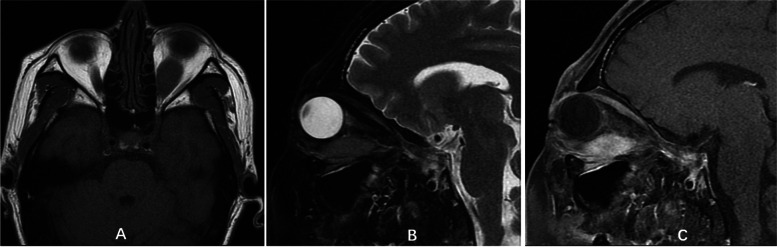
Orbital MRI. T1 weighted image shows the lesion is low signal (A, orbital horizontal scan); T2 weighted image shows medium signal (B, orbital sagittal scan); The lesion mass is significantly enhanced (C, orbital sagittal enhanced scan); Part lesion is adhesion to the inferior rectus muscle and optic nerve (B and C)
